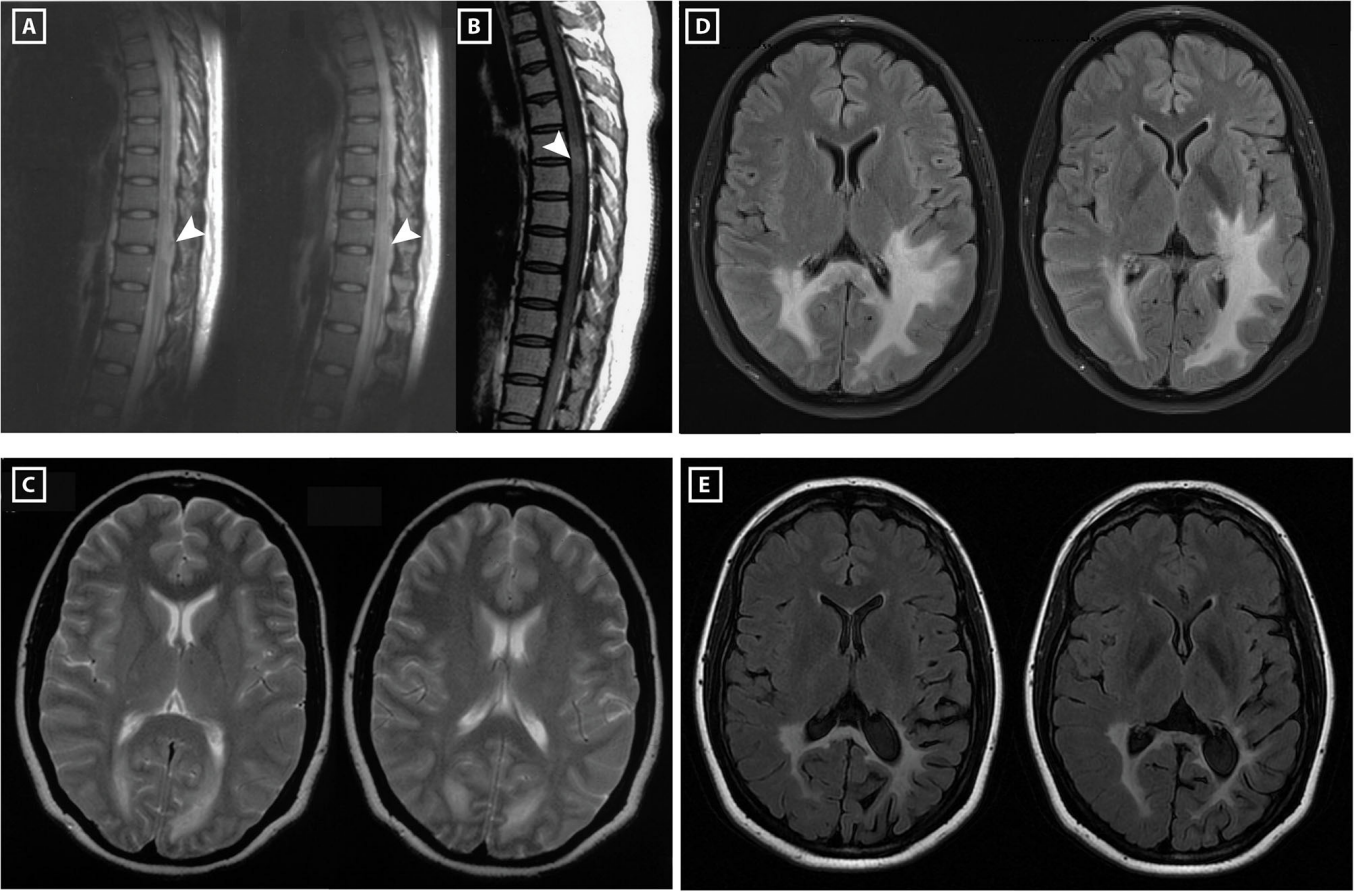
Spinal cord and brain MRI of the patient. In 2003, MRI of the spinal cord showed a hyper-intense lesion at T9–T11 levels (A), and gadolinium-enhanced T1-weighted MRI showed a hyper-intense lesion at T4–T5 levels (B). Her brain MRI revealed periependymal lesions around occipital horns of lateral ventricles (C). In 2009, brain MRI showed T2 hyper-intense lesions around occipital horns of both lateral ventricles extending into left temporal and occipital lobes, right occipital lobe and splenium of the corpus callosum (D). In 2017, brain MRI showed the white matter lesion reduced in size compared to the MRI performed in 2009 (E).
